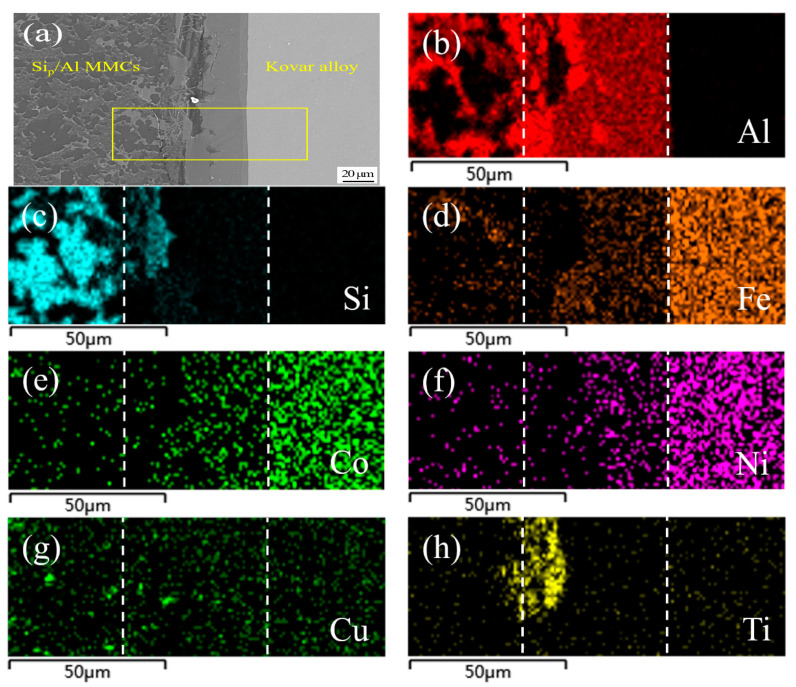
SEM images of typical region at the interface of 50 wt.% Si_p_/Al MMCs/Kovar made using Al-7.5Si-23.0Cu-2.0Ni-2.5Ti at 580 °C for 30 min and corresponding energy dispersive X-ray maps showing distribution of elements: (a) SEM image; (b–h) individual elemental mapping of Al, Si, Fe, Co, Ni, Cu, and Ti, respectively.
